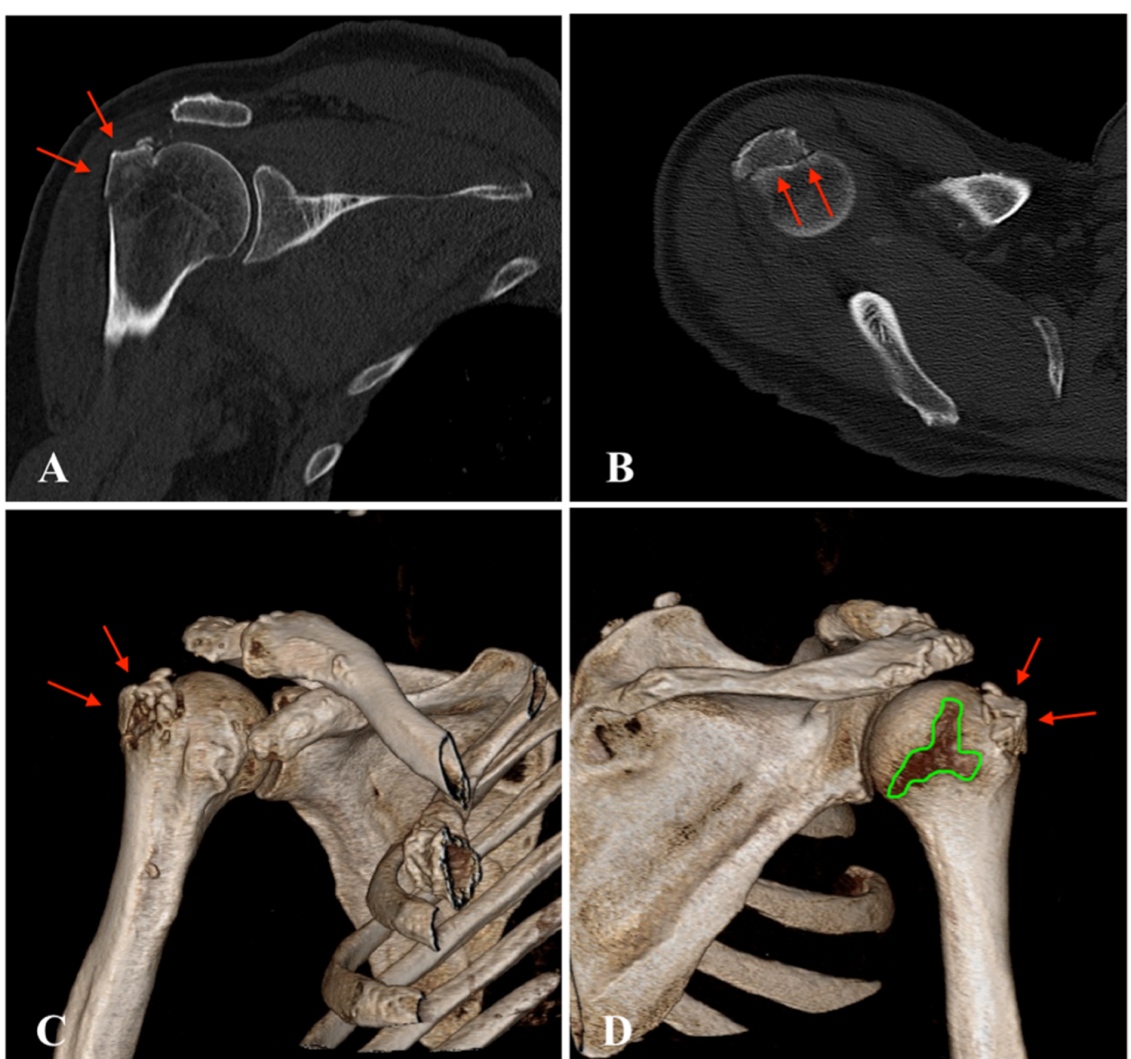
Computed tomography (CT) of the right shoulder after inferior dislocation: (A), coronal scan with greater tuberosity fracture (red arrows); (B), axial scan with greater tuberosity fracture (red arrows); (C), anterior 3D reconstruction with nearly undisplaced greater tuberosity fracture (red arrows); (D), posterior 3D reconstruction with nearly undisplaced greater tuberosity fracture (red arrows) and visible bare area of the humeral head (green area).
